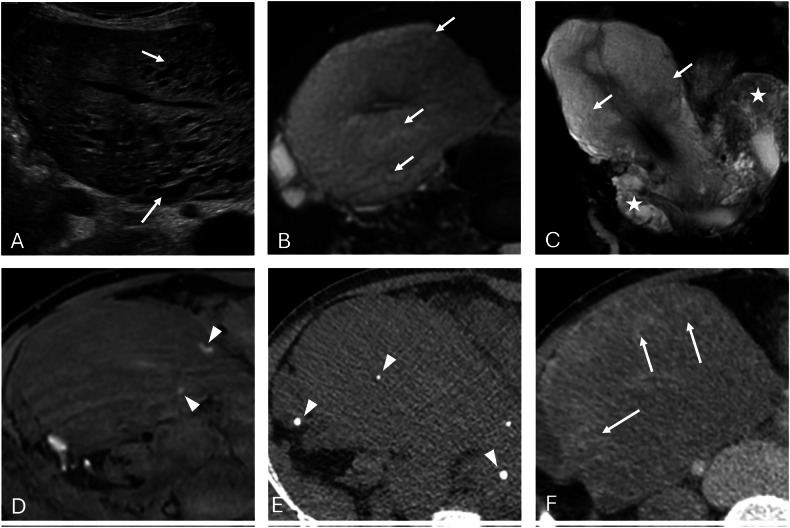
Multiple venous malformations of the gynaecological tract, bowel, soft tissues, and lower limbs in a 46-year-old patient with Klippel-Trénaunay syndrome and a PIK3CA mutation. A Suprapubic ultrasonography image shows a diffuse myometrial enlargement with multiple anechoic myometrial serpiginous structures (arrows). B Axial and C coronal T2-W fat-suppressed MR images show marked uteromegaly with a diffuse T2-hyperintense thickening of the myometrium and a sponge-like pattern (arrows). Note the similar enlargement of the adjacent affected bowel loops (stars). D Axial T1-W fat-suppressed MR image shows some scattered hyperintense areas (arrowheads) corresponding to focal thrombi within the venous malformation. E Axial non-contrast CT image shows scattered calcifications within the myometrium and bowel loops, corresponding to tiny phleboliths (arrowheads). F Axial post-contrast CT image shows minimal and delayed peripheral enhancement of the uterine venous malformation (arrows)
